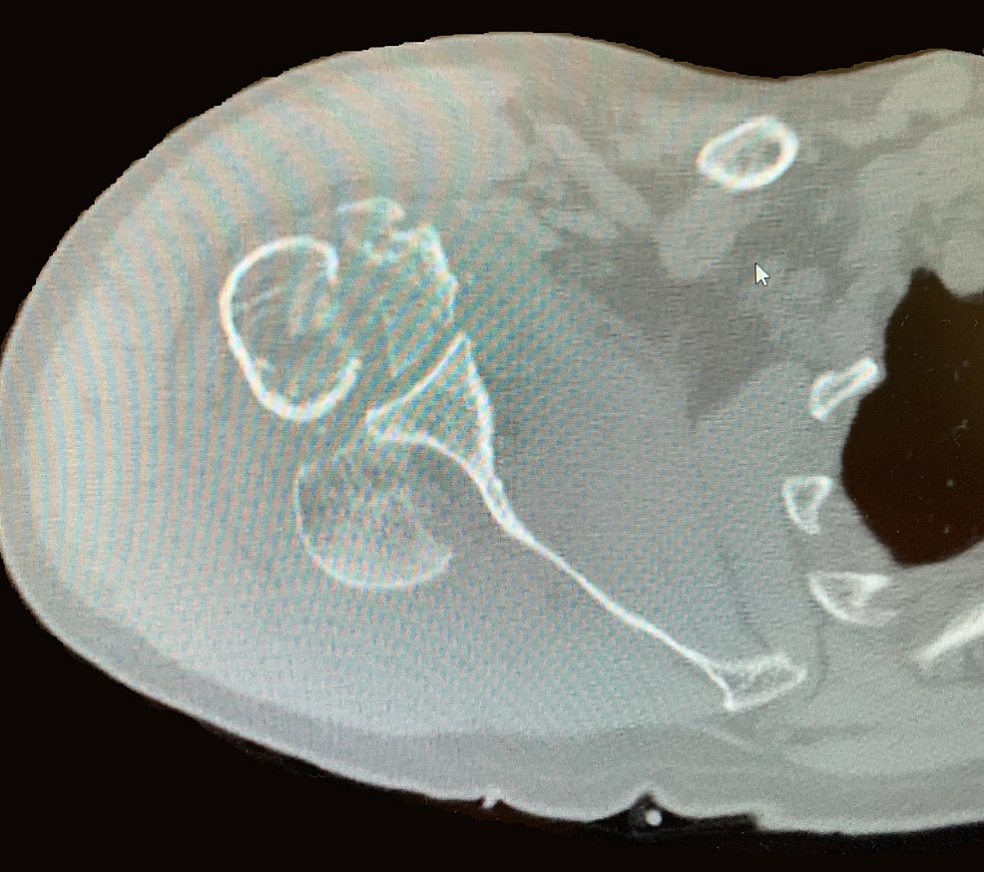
Axial view of preoperative CT scan of the right shoulder demonstrating a four-part proximal humerus (PH) posterior fracture-dislocation.
